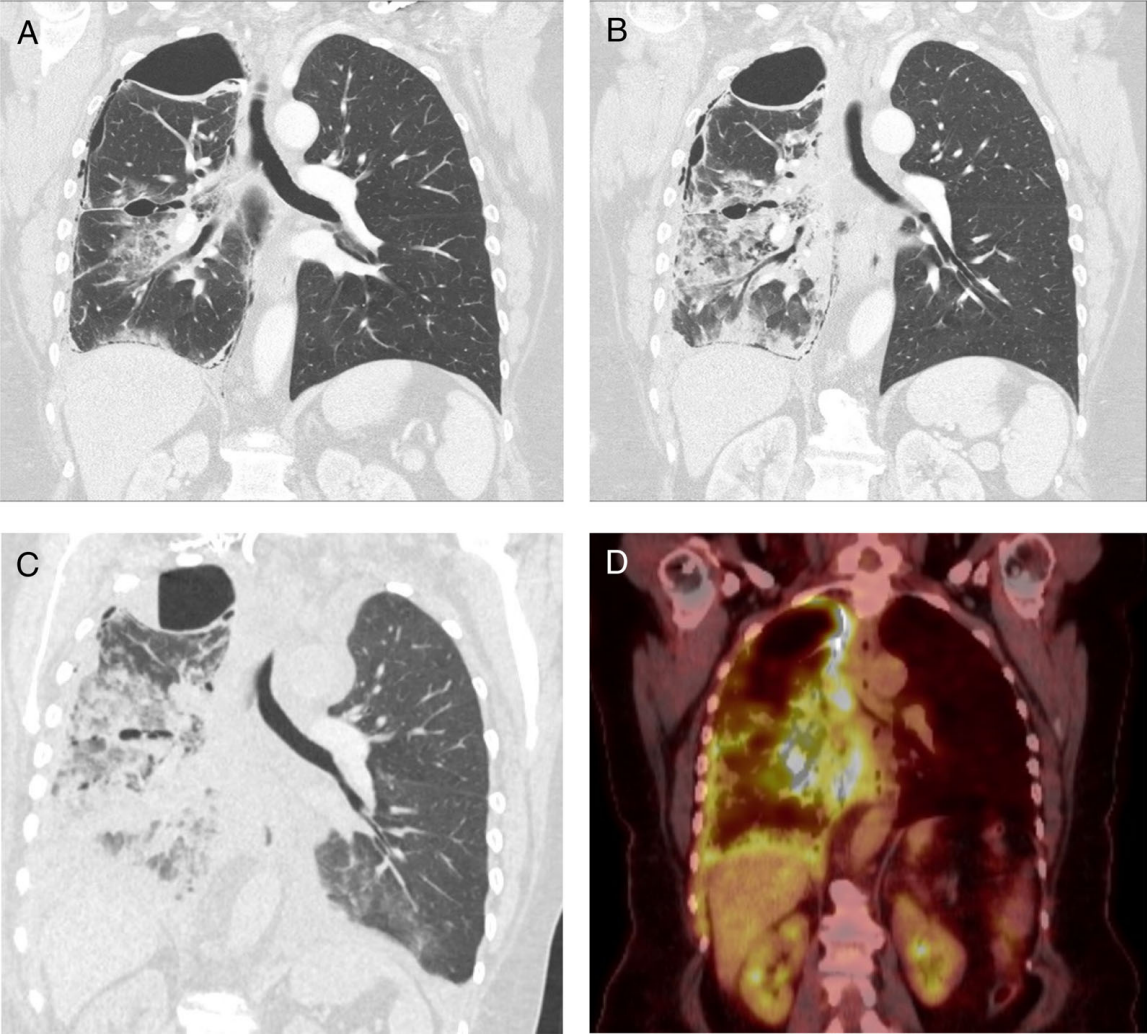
Evolution of pulmonary infiltrates in the right middle and lower lobes at six weeks (A), four months (B), and five months post video‐assisted thoracoscopy (VAT) biopsy (C). Positron emission tomography (PET) demonstrates these infiltrates are FDG‐avid (D).
